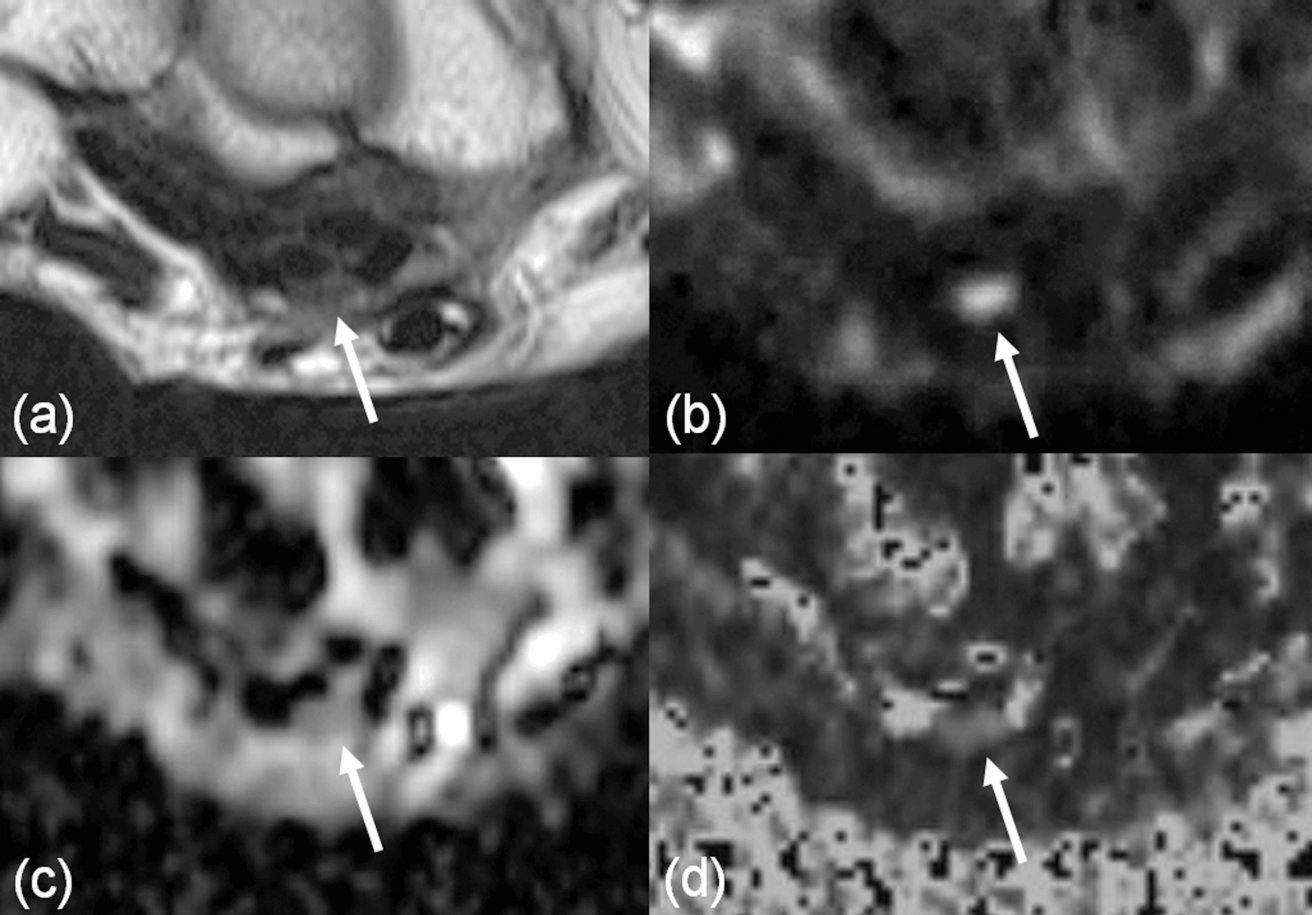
Diffusion tensor imaging of the wrist (NCV-normal case) FA: fractional anisotropy; ADC: apparent diffusion coefficient; NCV: nerve conduction velocity; T2WI: T2-weighted imaging; DWI: diffusion-weighted imaging A 68-year-old male patient presented with numbness in the fingertips of the left hand and was initially suspected of having carpal tunnel syndrome, but NCV was normal. Axial images of the left wrist at the level proximal to the pisiform bone are shown: (a) T2WI (4-mm thickness), (b) DWI (b = 1400, 4-mm thickness), (c) ADC map, and (d) FA map. The arrow indicates the median nerve. The ADC was 0.739 × 10⁻³ mm²/s, and the FA was 0.480
